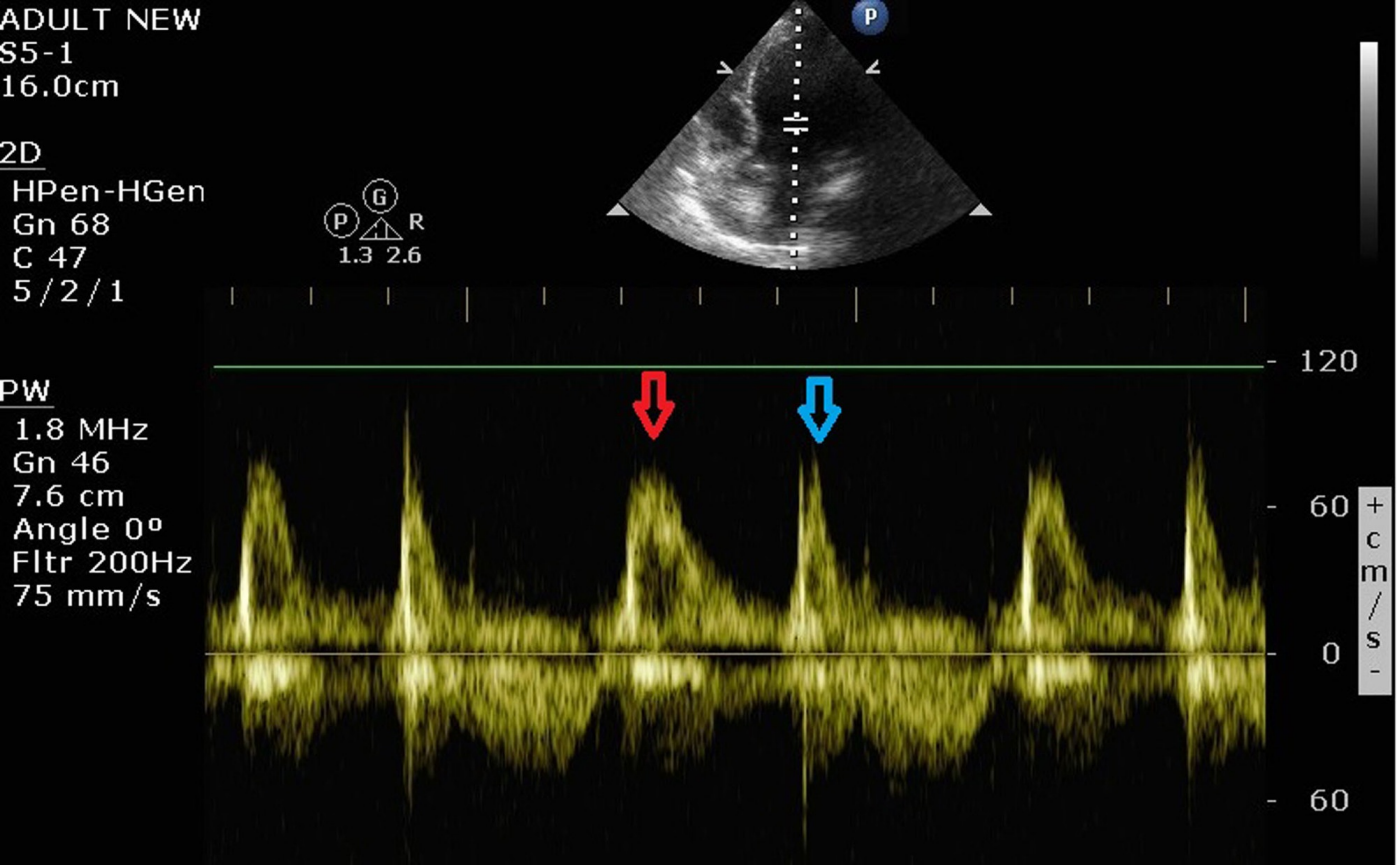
Pulsed wave Doppler revealing a diastolic pattern of normal sinus rhythm Red arrow shows early diastolic E wave and blue arrow late diastolic A wave.
